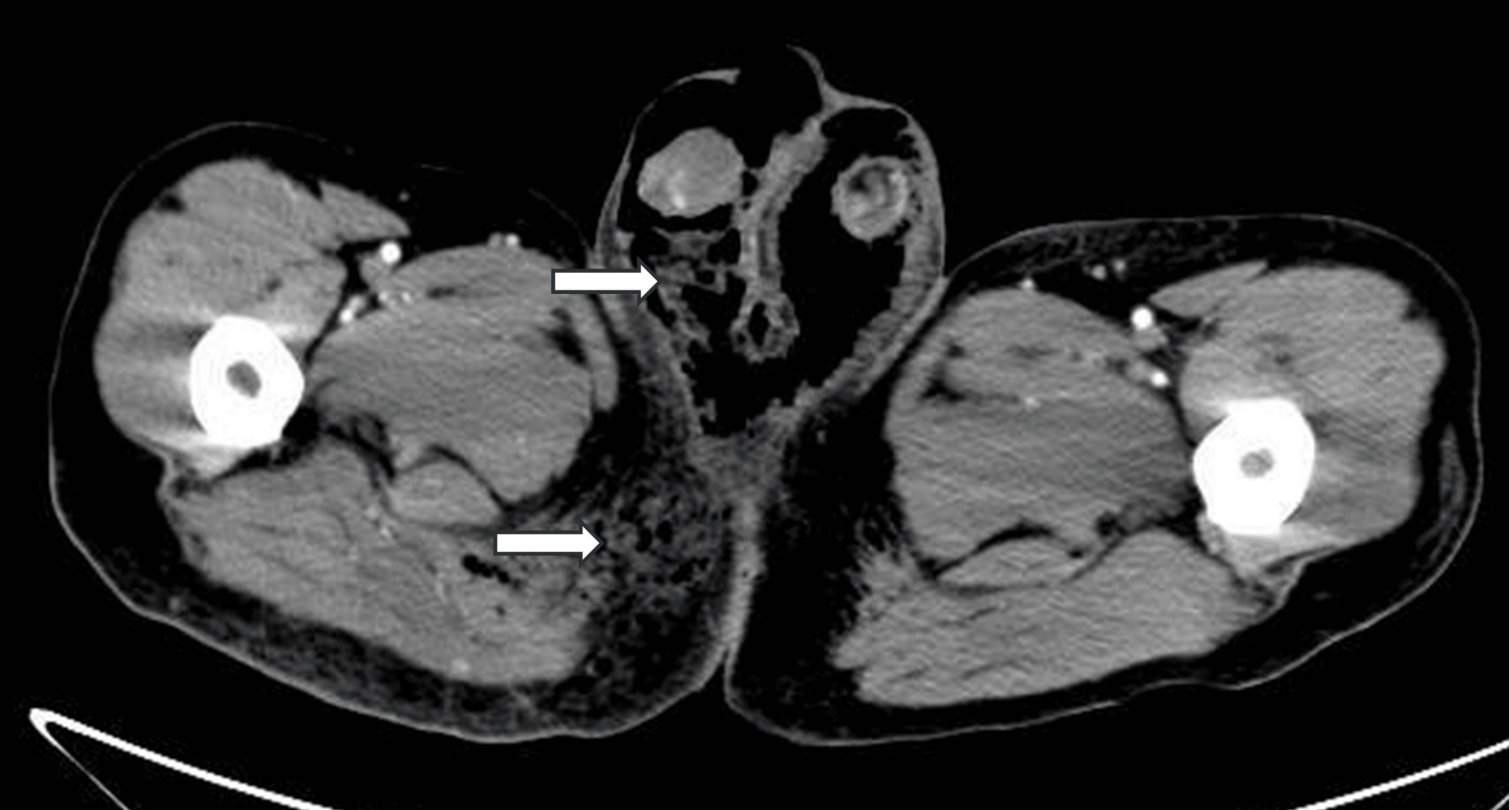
Pelvic CT of the left lower abdominal wall has right perineum infection.
